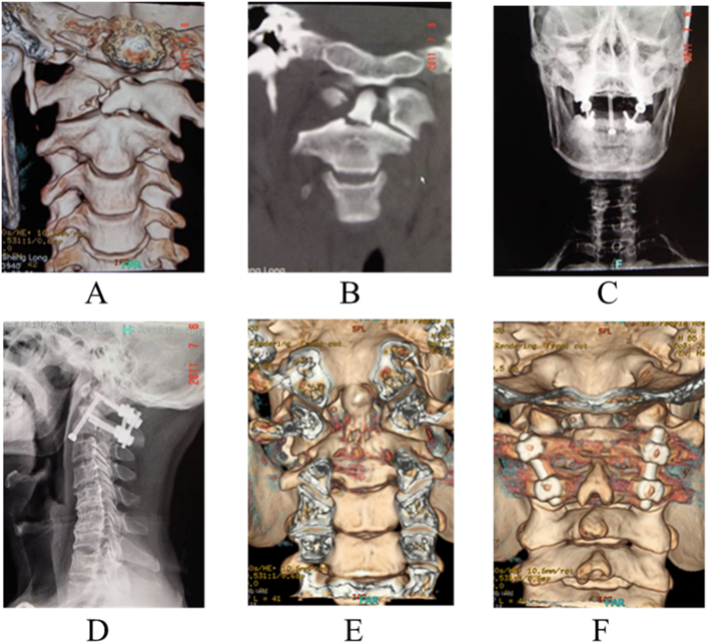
A 36-year-old male patient was admitted to our hospital due to neck pain and restricted neck motion as well as incomplete limbs paralysis from traffic accidents. Cervical spine 3D-CT scanning (A) and CT scanning in the coronal plane (B) showed that this patient suffered Jefferson combined with type II odontoid fractures. The patient underwent the posterior atlantoaxial pedicle screw internal fixation under general anesthesia (C, D). At 12 months of follow-up, the 3D-CT scanning showed that the fracture was healed and the graft was fused (E, F).
